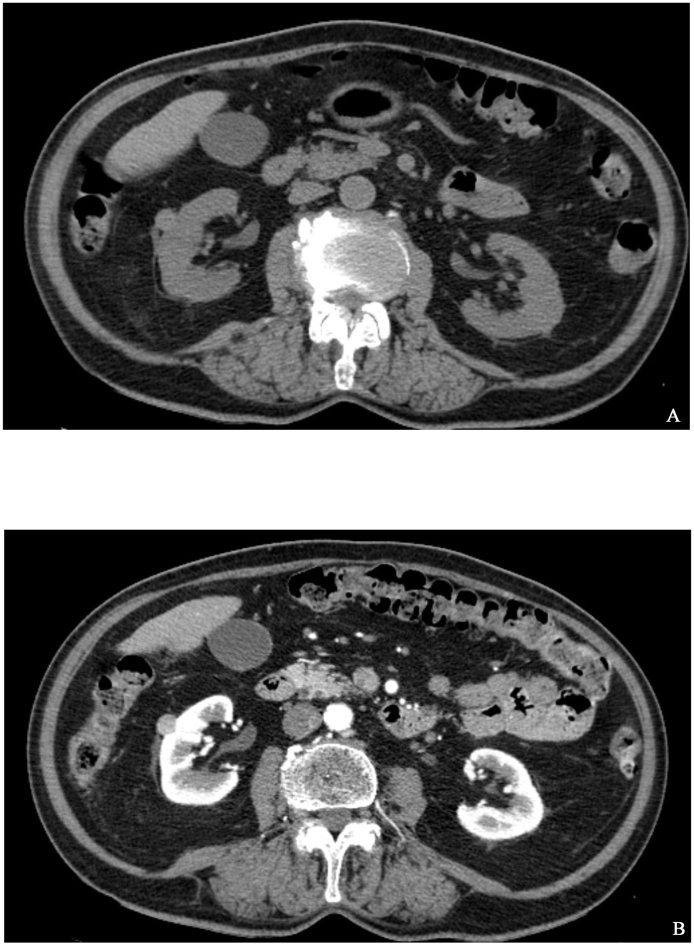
Abdominal CT scans. A. Non-enhanced CT shows subcapsular mass in right kidney; B.) CT with contrast, arterial phase, shows well-circumscribed, high-density solid lesion with enhancement.
